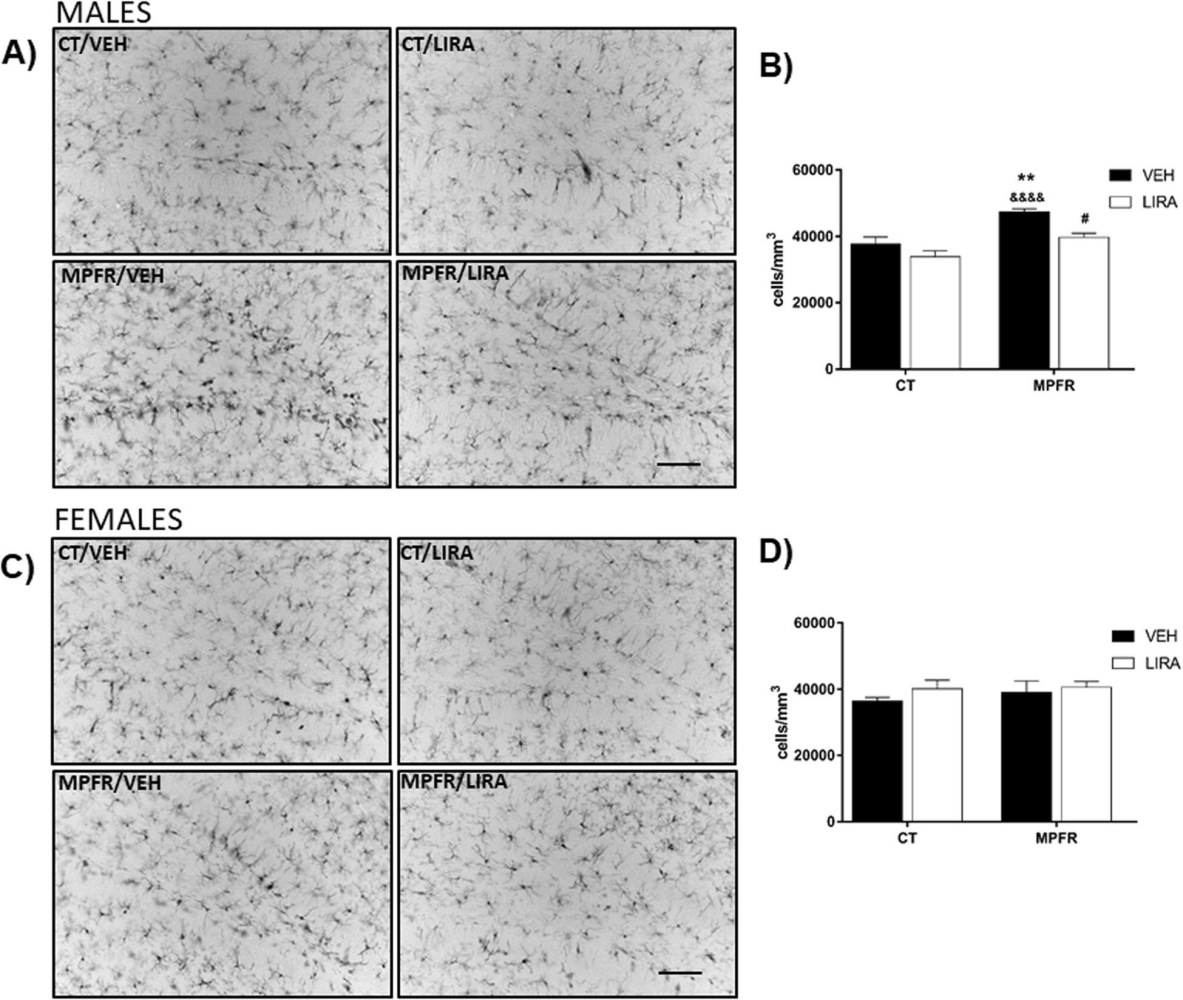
a Representative images of Iba-immunoreactivity in the dentate gyrus of male pups. Scale bar, 20 μm. b Quantification of Iba-1 immunoreactivity in the dentate gyrus in male pups. c Representative images of Iba-immunoreactivity in the dentate gyrus of female pups. Scale bar, 45 μm. d Quantification of Iba-1 immunoreactivity in the dentate gyrus in female pups. CT/VEH, control pups treated with vehicle; CT/LIRA, control pups treated with liraglutide; MPFR/VEH, food-restricted pups treated with vehicle; MPFR/LIRA, food-restricted pups treated with liraglutide. Data are mean + SEM. (*p* < 0.01 compared to CT/VEH; p* < 0.001 compared to CT/VEH; ^&&^*p* < 0.01 compared to CT/LIRA; ^&&&&^*p* < 0.0001 compared to CT/LIRA; ^#^*p* < 0.05 compared to MPFR/VEH; ^##^*p* < 0.01 compared to MPFR/VEH)
